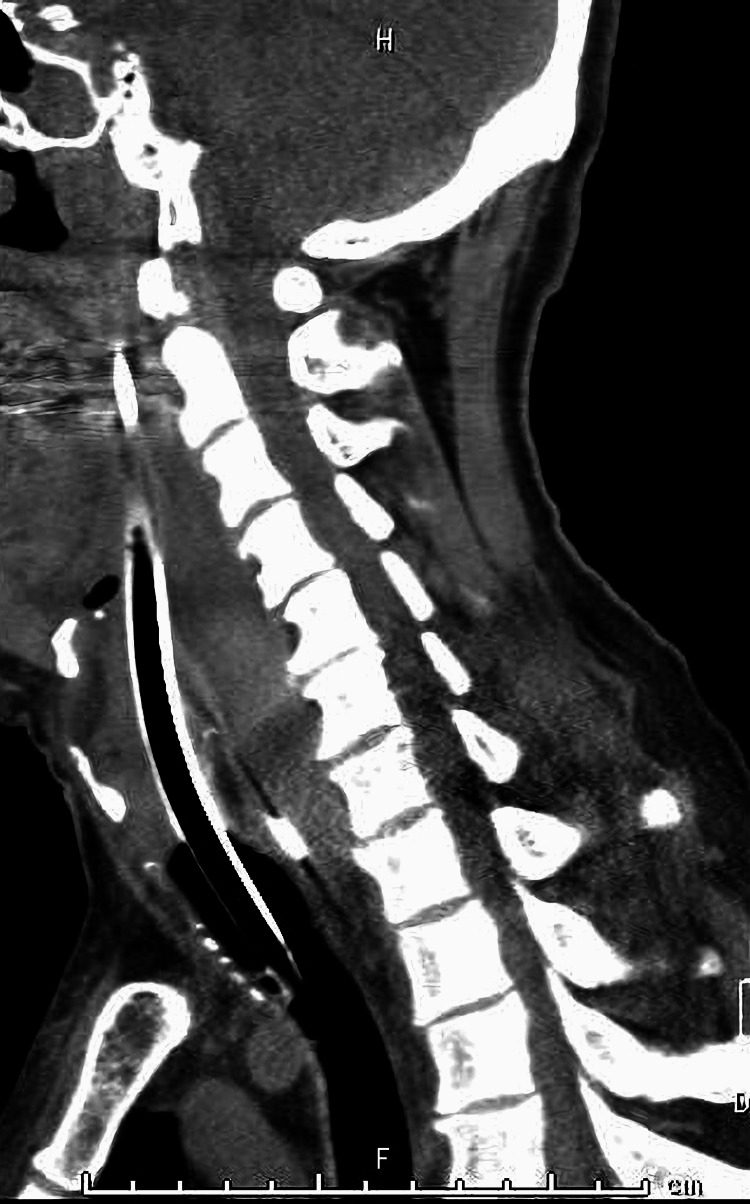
Contrast-enhanced CT scan on the 15th day of hospitalization Compared to the initial examination, the hematoma has shrunk.
